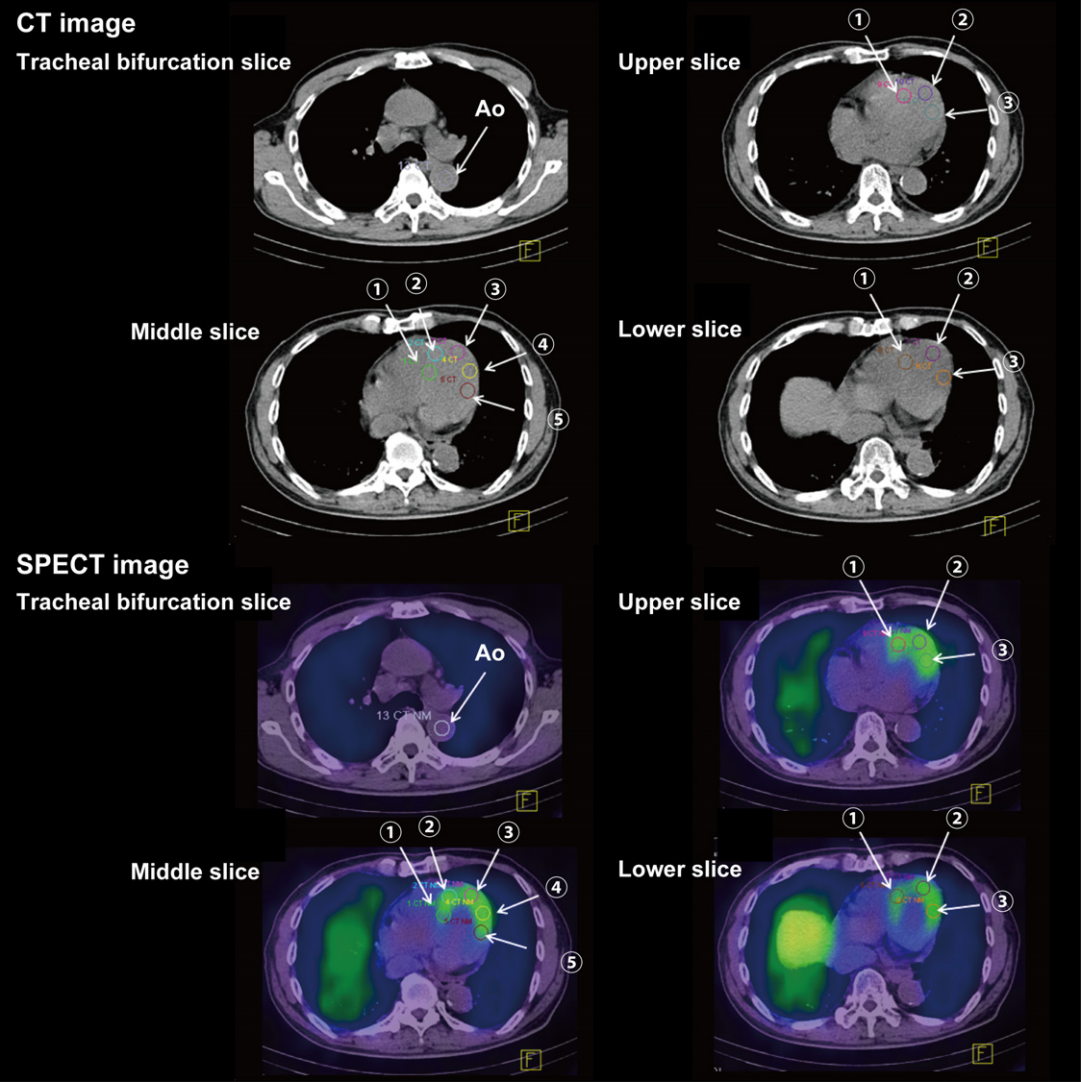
An example of manually defined ROIs on CT images and anatomical localization of ROIs on SPECT/CT fusion images. First, myocardial ROIs and an aorta ROI were placed on the CT images. Next, anatomical localization of.ROIs on a SPECT/CT fusion images were checked by the operator before the analysis.
